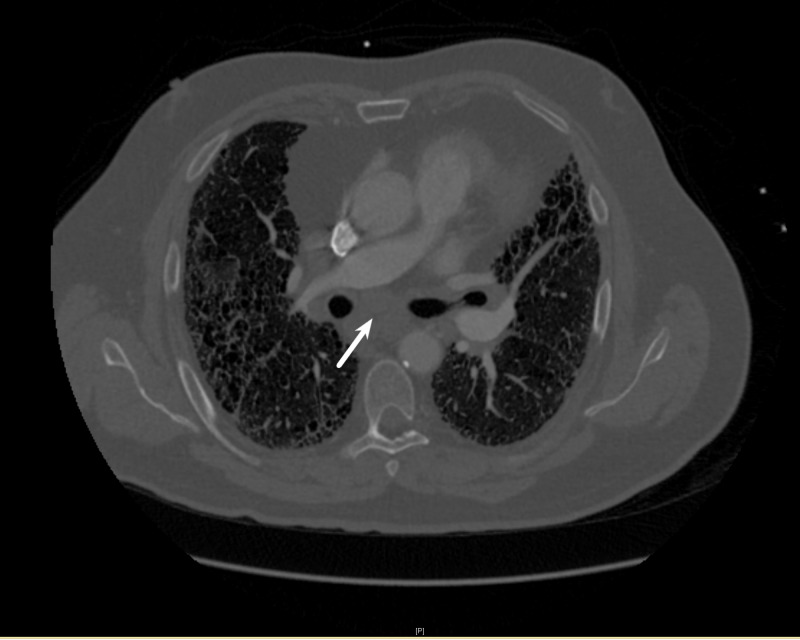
CT angiography of the chest showing subcarinal mass (arrow)
